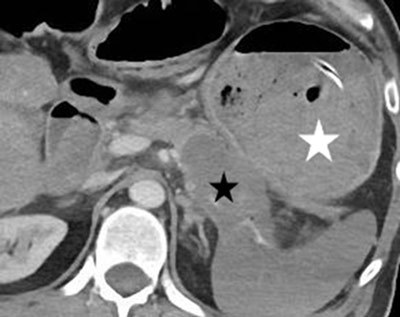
Axial contrast-enhanced CT image: the pancreatic neoplasm (black star) and the almost entirely clotted gastric lumen (white star).
